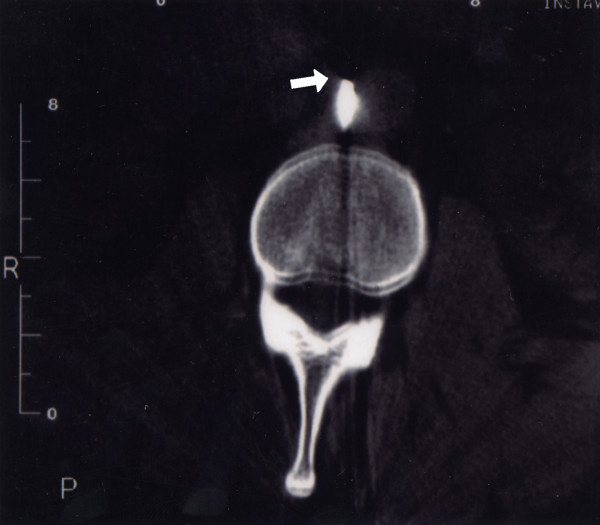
CT scan demonstrating tip of screwdriver abutting the abdominal aorta.
